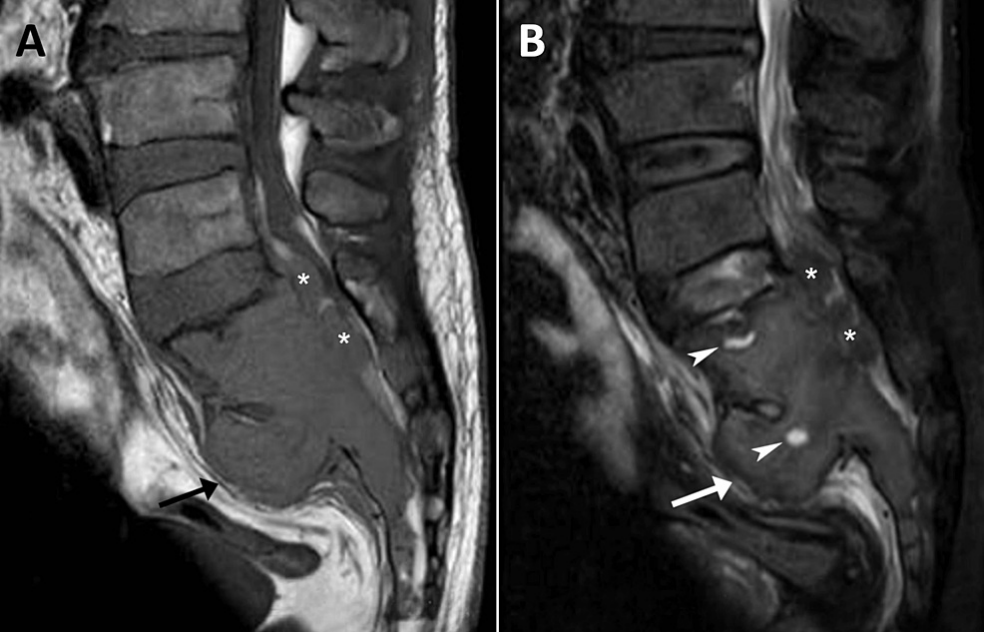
Lumbar spine magnetic resonance imaging, T1-weighted (A) and T2-weighted (B) sagittal views. There is a large, expansile bone lesion in the sacral ala and bodies S1-S3, which is mildly hypointense on T1 and intermediate signal intensity on T2, with small areas of hyperintensity on the T2-weighted view (B, arrowheads), suggestive of cystic spaces. There is anterior cortical destruction at S2 with soft-tissue penetration into the pre-sacral space (arrow). Also noted is cortical destruction and extension into the sacral spinal canal at the S1 and S2 levels (*).
